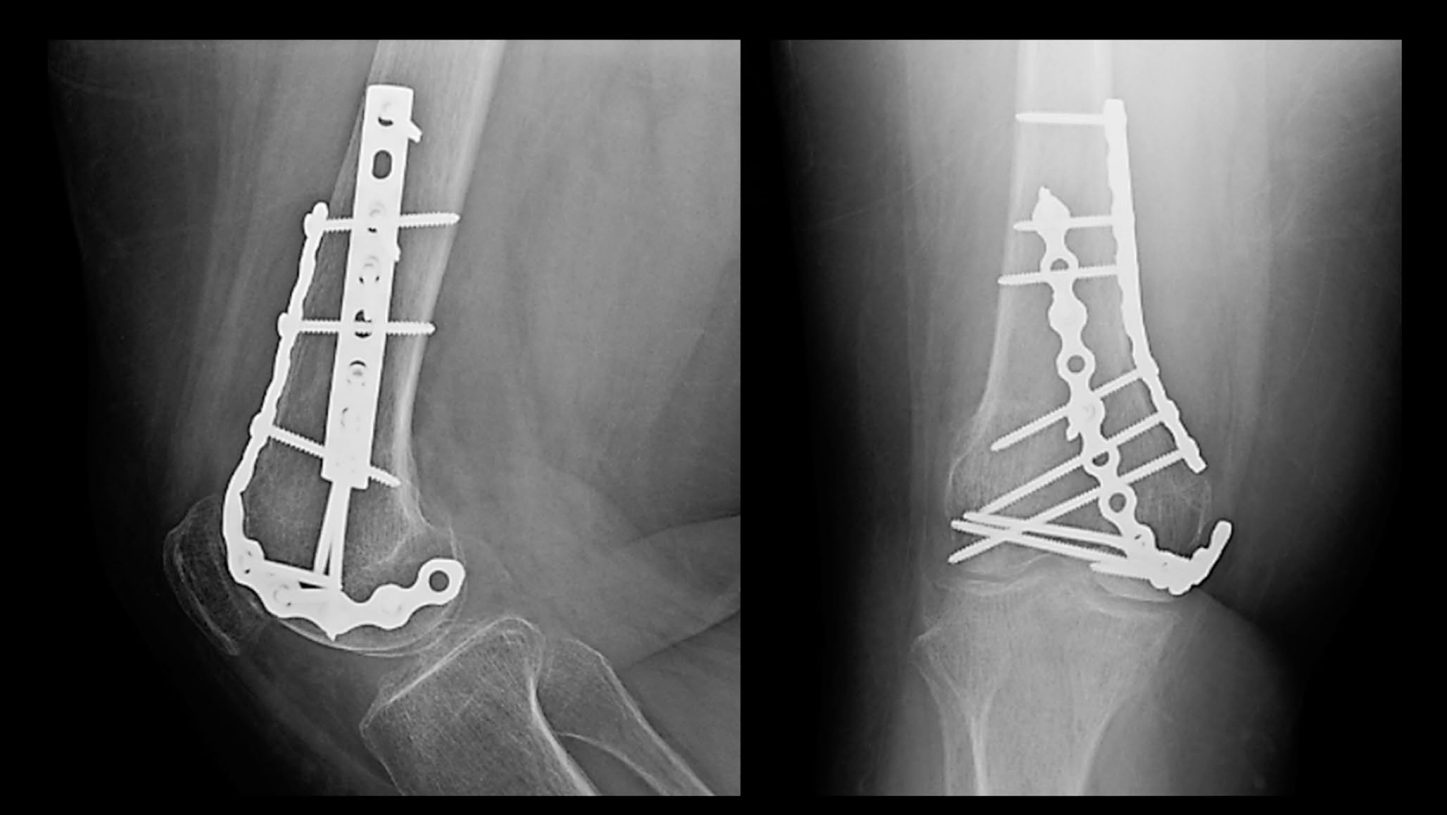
Final follow-up anteroposterior and lateral radiographs with healed fracture at 6 months postoperative
